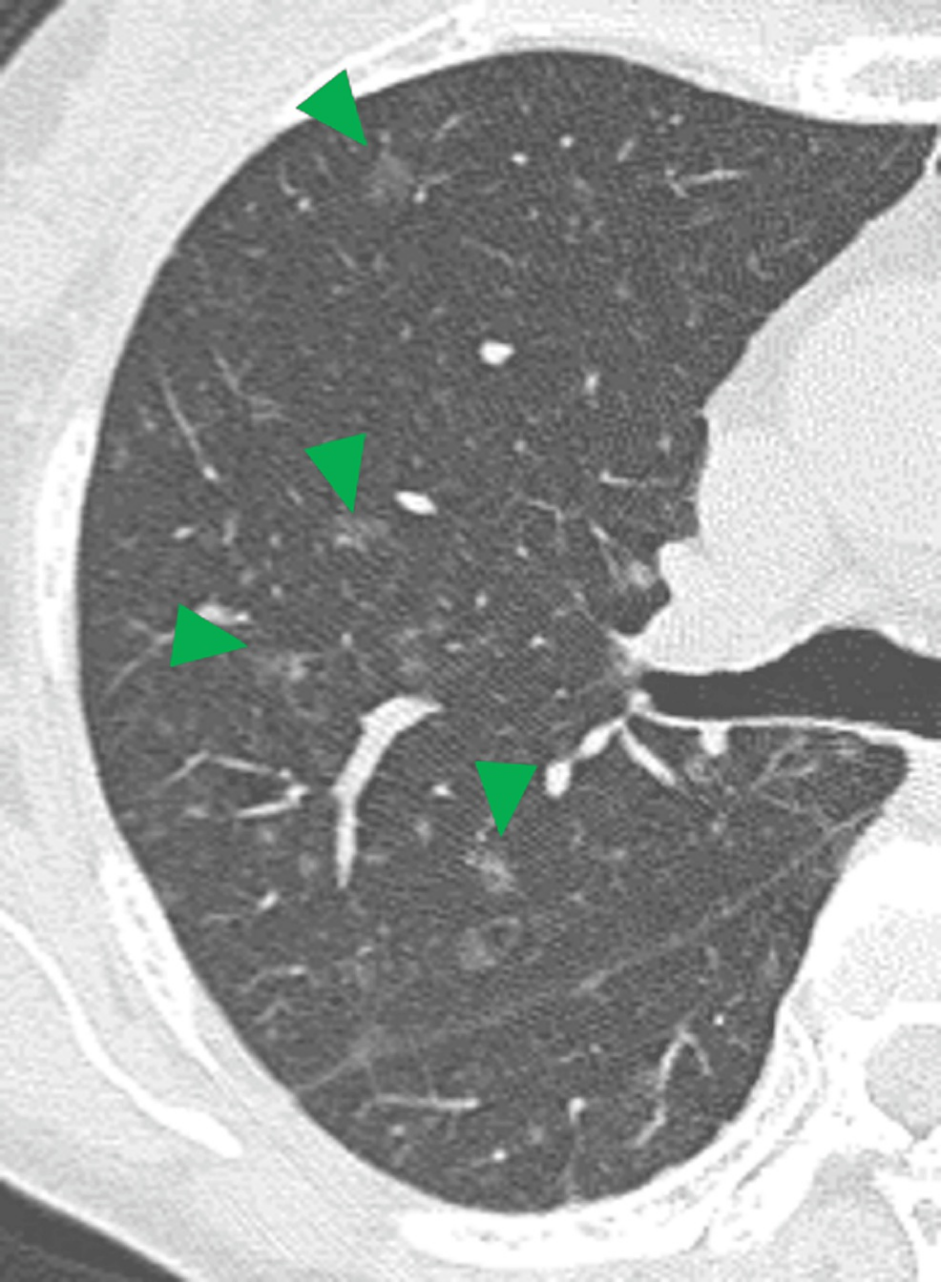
Chest CT scan shows multiple GGOs in both lungs (green arrowheads). GGOs = ground-glass opacities
